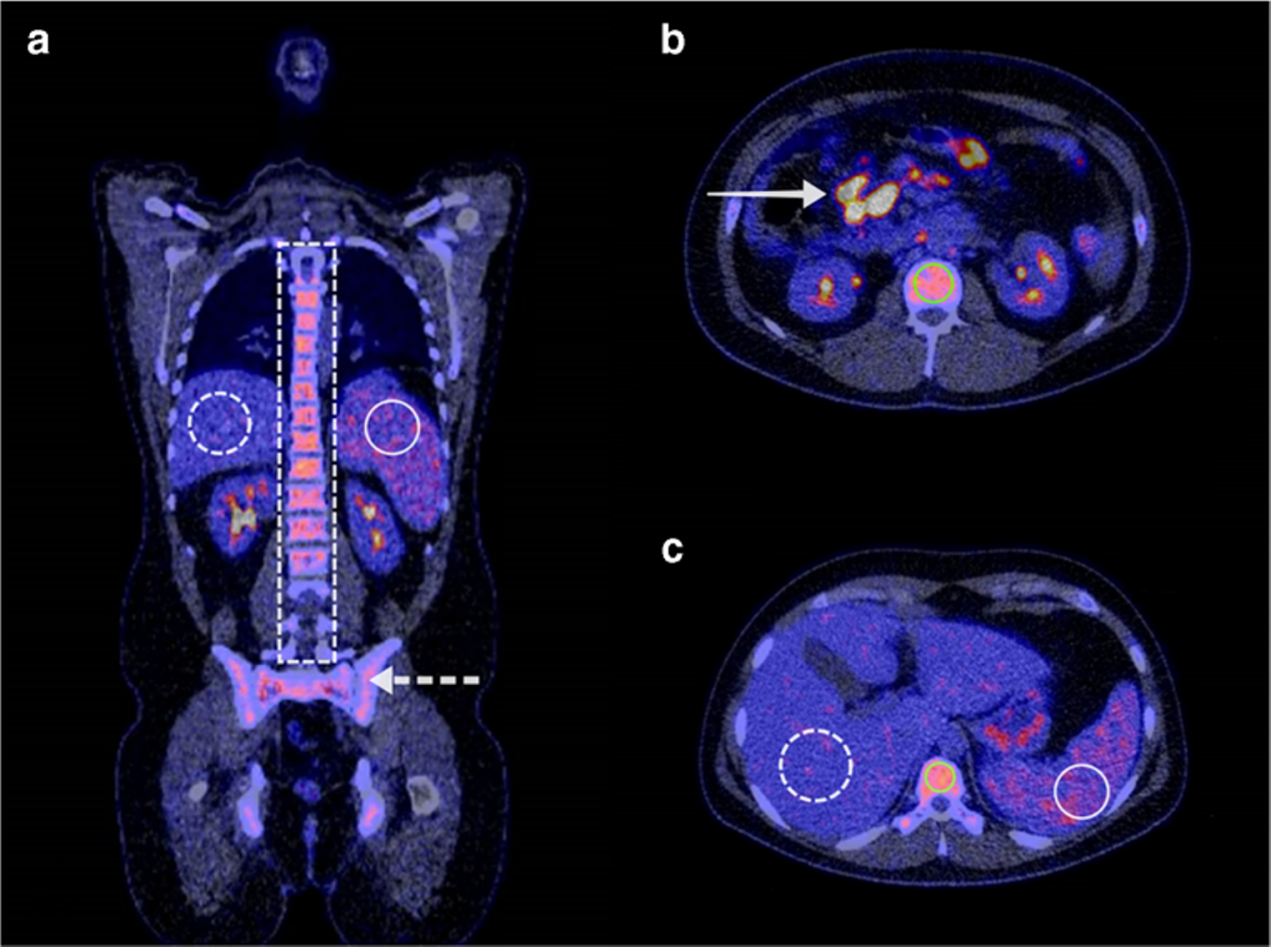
A 26-year-old man with no previous medical history presented at the hospital with a fever of 39 °C and watery diarrhea after a holiday to South America. A complete physical exam and a thoracic X-ray showed no abnormalities. His leukocyte count was 3.8 × 10^9^/L, and his CRP level was 165 mg/L. Blood cultures were positive for *Salmonella* species. Antibiotic treatment was initiated with ceftriaxone. Because his clinical condition did not improve, FDG-PET/CT was performed to identify the focus of infection. FDG-PET/CT showed diffuse high FDG uptake in the bone marrow, including the spinal vertebrae (A, dashed white rectangle; B, C, green circle) and pelvis (A, dashed white arrow) with a BLR of 1.40. FDG uptake in the spleen (A, C, white circle) was also higher than in the liver (A, C, dashed white circle) with an SLR of 1.26. Apart from mesenteric lymphadenitis (B, white arrow), no other infection focus was found. Antibiotic therapy was continued, and the patient was discharged 1 week after FDG-PET/CT in good clinical condition
